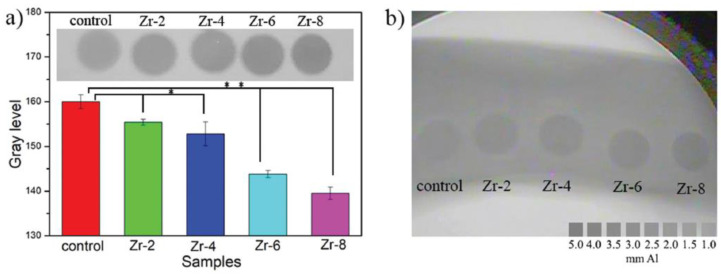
(a) X-ray photograph of ZrO_2_ short fiber (2–8 wt.%)-incorporated CPCs and the analyzed grey level; (b) X-ray photograph of cements in a bone mold. * *p* < 0.05 vs. control.  *p* < 0.01 vs. control [94].
